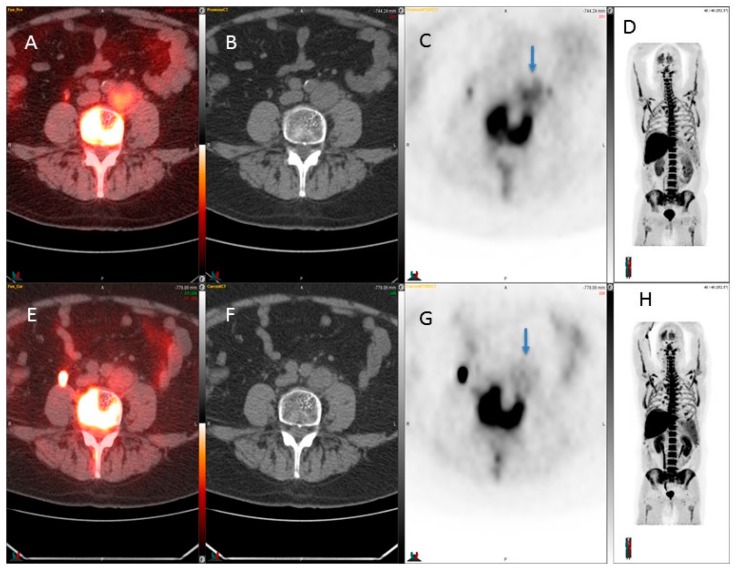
^18^F-FLT used for detection of retroperitoneal lymph node metastases before and after chemotherapy. A weak response was seen while the SUVmax value decreased from 4.1 to 2.1 in the left common iliac node (arrows in C and G). Upper row, baseline: from the left fusion image (A), CT image (B), ^18^F-FLT PET image (C), and MIP image (D); lower row, after 4 week therapy: from the left fusion image (E), CT image (F), ^18^F-FLT PET image (G), and MIP image (H). Color bar range from 0 to 5.
